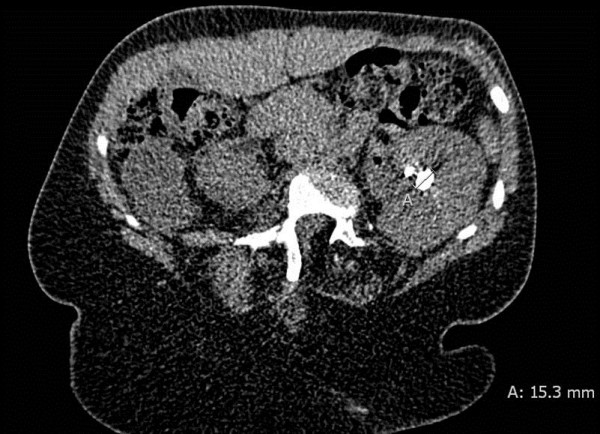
The pre-operative CT-scan revealed a 15.4 mm stone in the lower calix of the left kidney. Metal wires in the renal parenchyma were adjacent to the stone. Coiling material in the collecting system or within the stone was not identified.
